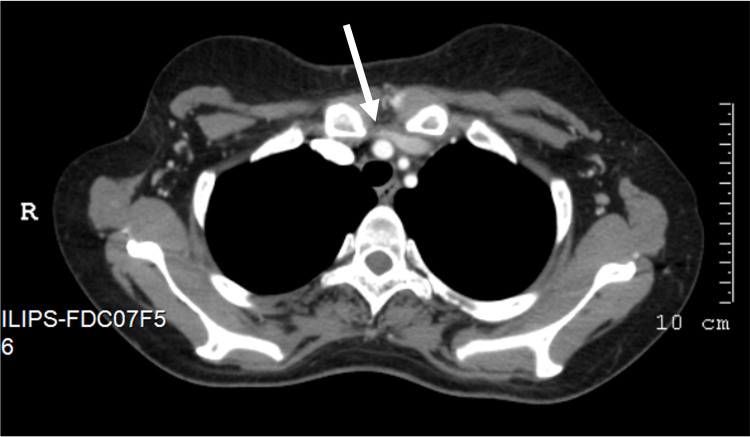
Stenosis of the left brachiocephalic venous trunk and thoracic duct (arrow) associated with adenopathies (CT chest axial plane).
